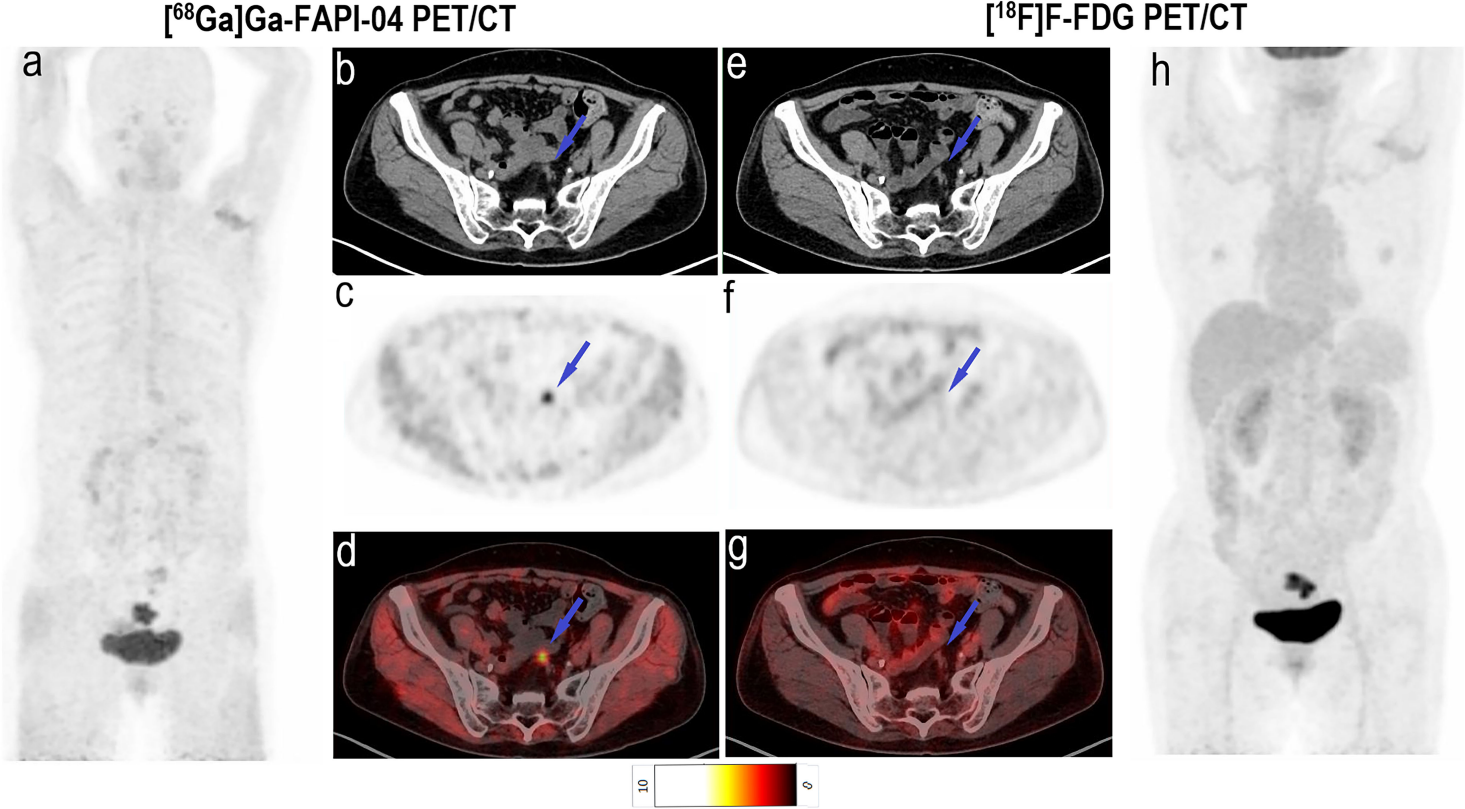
Images of a 64-year-old woman with rectal cancer for staging. (A) Mean intensity projection images of [^68^Ga]Ga-FAPI-04 PET/CT scan. (B–D) Axial CT, PET and fused images of [^68^Ga]Ga-FAPI-04 PET/CT scan. (E–G) Axial CT, PET and fused images of [^18^F]F-FDG PET/CT scan. (H) Mean intensity projection images of [^18^F]F-FDG PET/CT scan. Pelvic peritoneal carcinoma is distinctly observed in [^68^Ga]Ga-FAPI-04 PET/CT images (blue arrows in b–d) because of intensive tracer uptake (SUV_max_ 5.5). Conversely, little [^18^F]F-FDG (SUV_max_ 1.1) uptake results in the small lesion being hardly detectable (blue arrows in E–G).
